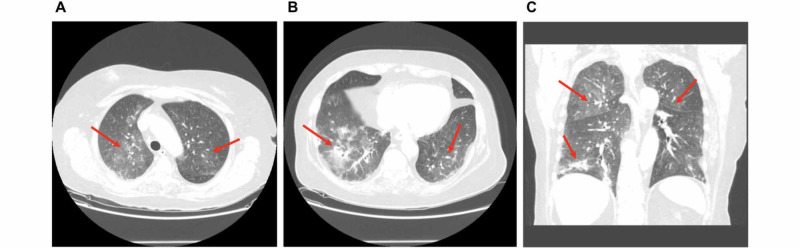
Various CT scan views display (A) ground-glass opacities in the bilateral upper lobes (axial view), (B) ground-glass opacities in the bilateral lower lobes (axial view), and (C) ground-glass opacities in the bilateral upper lobes in addition to linear atelectasis in the right lower lobe (coronal view).
